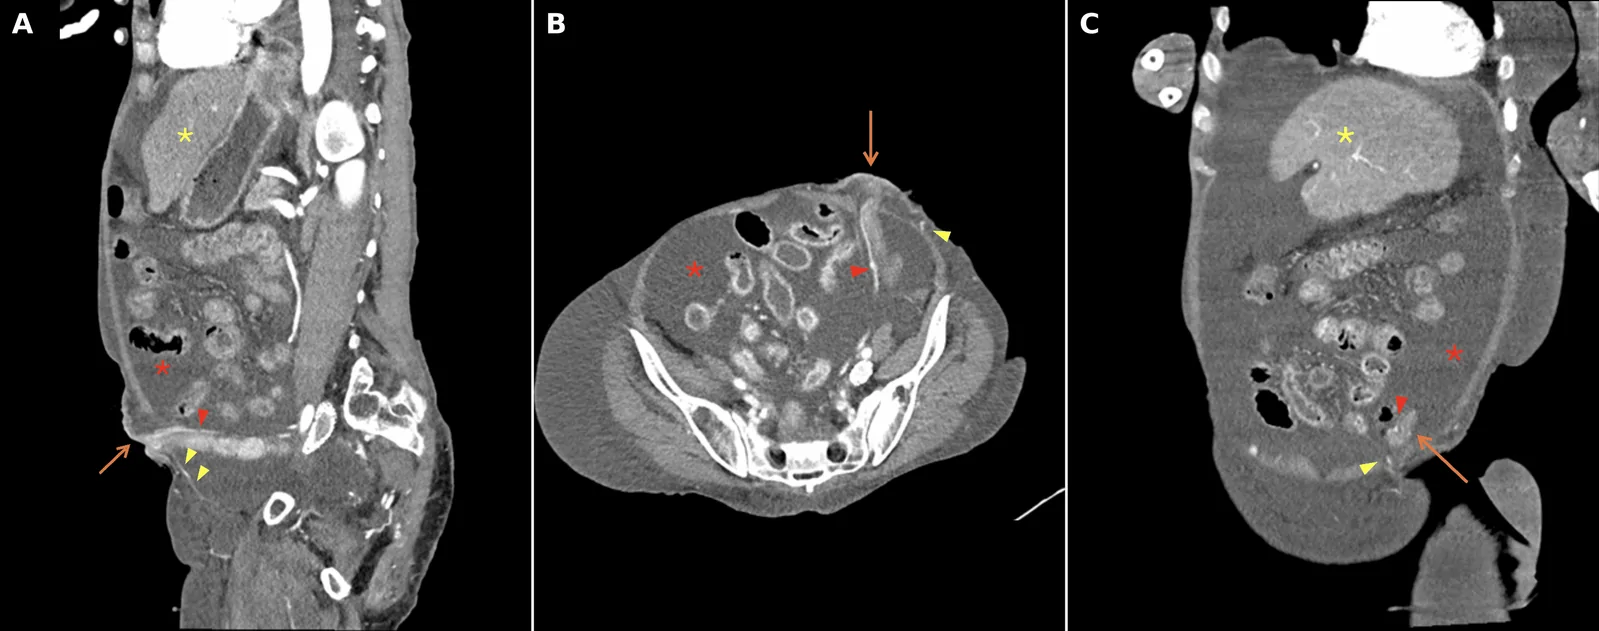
Sagittal, axial, and coronal CT images (A, B, and C, respectively) demonstrate a loop colostomy in the left lower quadrant (orange arrow). Engorged small veins along the ostomy conduit (red arrowheads) communicate with systemic veins within the abdominal wall near the ostomy exit (yellow arrowheads), forming peristomal varices. Additional findings include large-volume ascites (red asterisks) and cirrhotic liver morphology (yellow asterisks). Image quality was limited by motion and upper-extremity beam-hardening artifacts related to the patient’s clinical condition.
